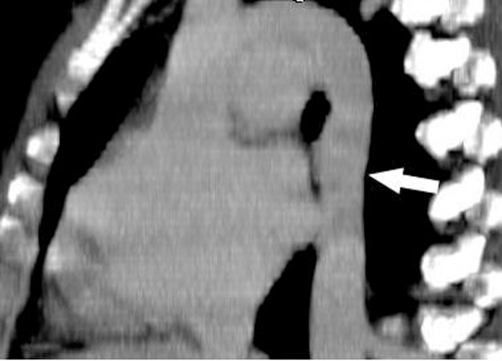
CT axial image that demonstrates enlarged azygos vein, draining into the superior vena cava (Arrow).
